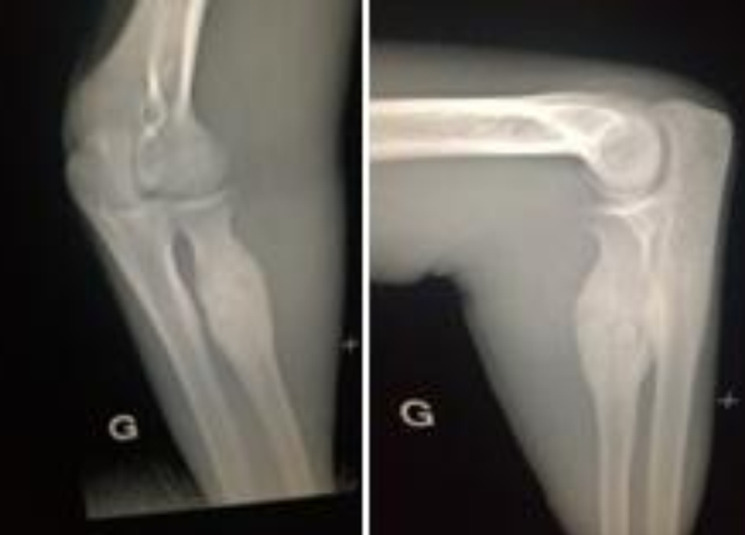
radiographie de face et de profil montrant l´image en cocarde sur l´extrémité supérieure du radius côté gauche
